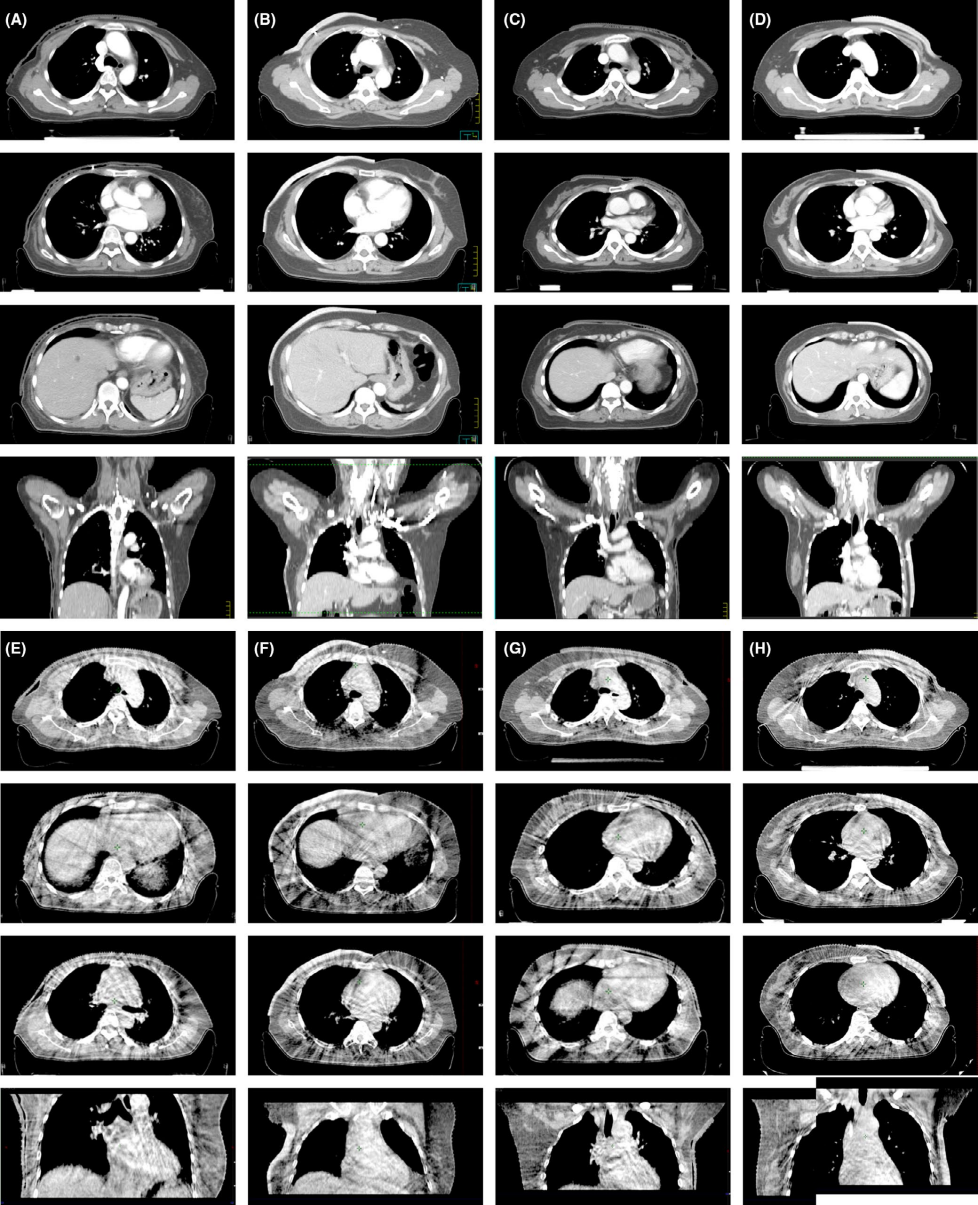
Examples of conventional bolus and 3D‐printed bolus CT (A–D) and CBCT images (E–H). A and E were right‐sided breast with the conventional bolus, B and F were right‐sided breast with the 3D‐printed bolus, C and G were left‐sided breast with the conventional bolus, and D and H were left‐sided breast with 3D printed bolus
